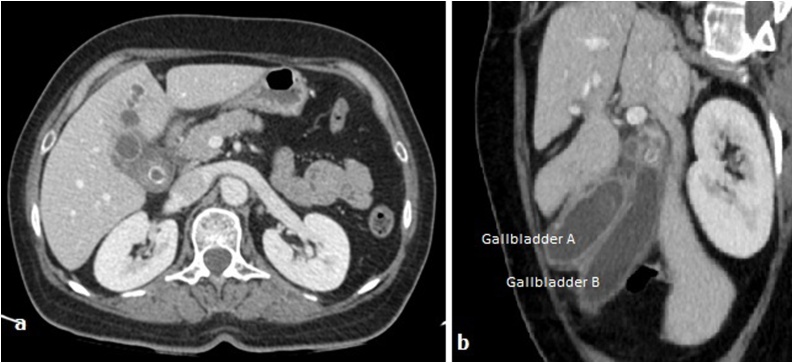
(a) Computed tomography scan showing lithiasic cholecystitis complicated by abscess in segment VI of the liver. (b) The sagittal 2D reconstruction displaying two gallbladders (A and B) with a common peritoneal coat.
